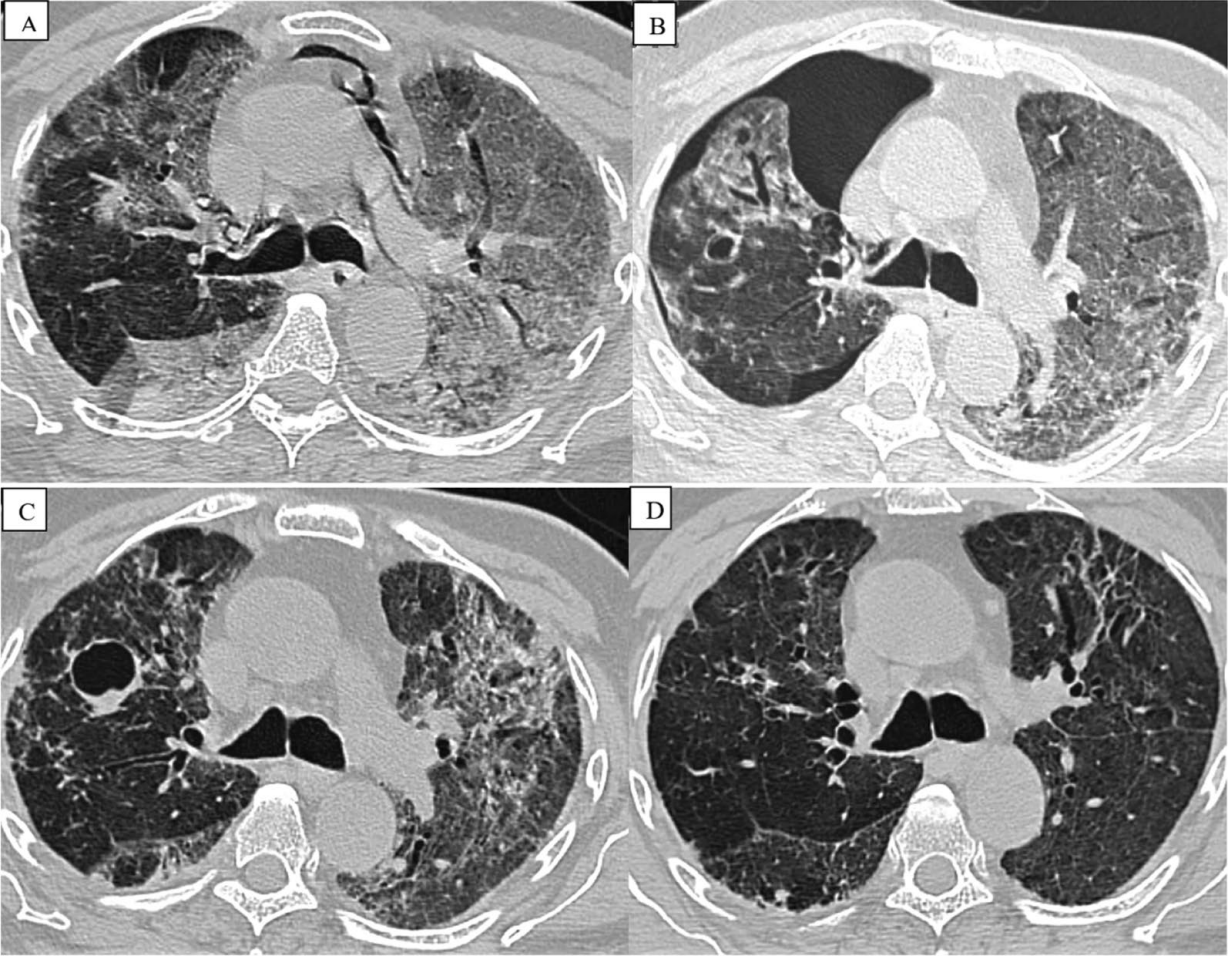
A 62-year-old COVID-positive man with acute dyspnea and cough was brought to the hospital. The patient was suffering from acute ARDS. He was admitted to the intensive care unit and placed on mechanical ventilation. The first HRCT scan A indicated significant consolidation and ground glass opacities in both lungs with pneumomediastinum. He developed pneumothorax on repeat CT B after 7 days of mechanical ventilation. The patient's clinical state improved over time, and a 20-day CT scan C revealed complete resolution of the pneumothorax and pneumomediastinum, but a new cavity had developed in the anterior portion of the right lung. Patient followed up after 3 months D revealed complete resolution of cavity with mild residual fibrosis in both lungs
